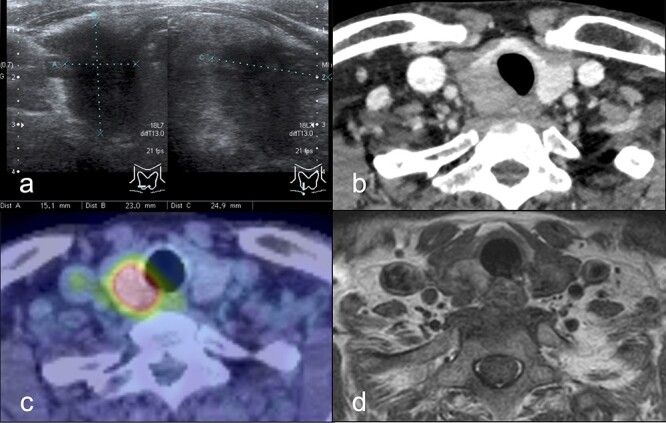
(a) Cervical ultrasound examination: a hypoechoic nodule with indistinct borders and irregular margins (15 × 23 × 25 mm) was found at the inferior pole of the right lobe. (b) Dynamic CT scan: a mass lesion (18 × 24 × 26 mm) was found in the inferior pole of the right lobe thyroid gland without contrast effect in equilibrium phase. (c) PET/CT scan: FDG hyperaccumulation was observed in a nodule (~25 mm diameter) at the inferior pole of the right lobe of the thyroid gland. (d) MRI scan: A mass (30 mm diameter) with mildly high signal on Dixon-T2-weighted imaging was found at the inferior pole of the right lobe of the thyroid gland, and tracheal invasion. Extracapsular invasion was suspected. Abbreviations: CT, computed tomography; PET, positive emission tomography; FDG, fluorodeoxyglucose; MRI, magnetic resonance imaging.
